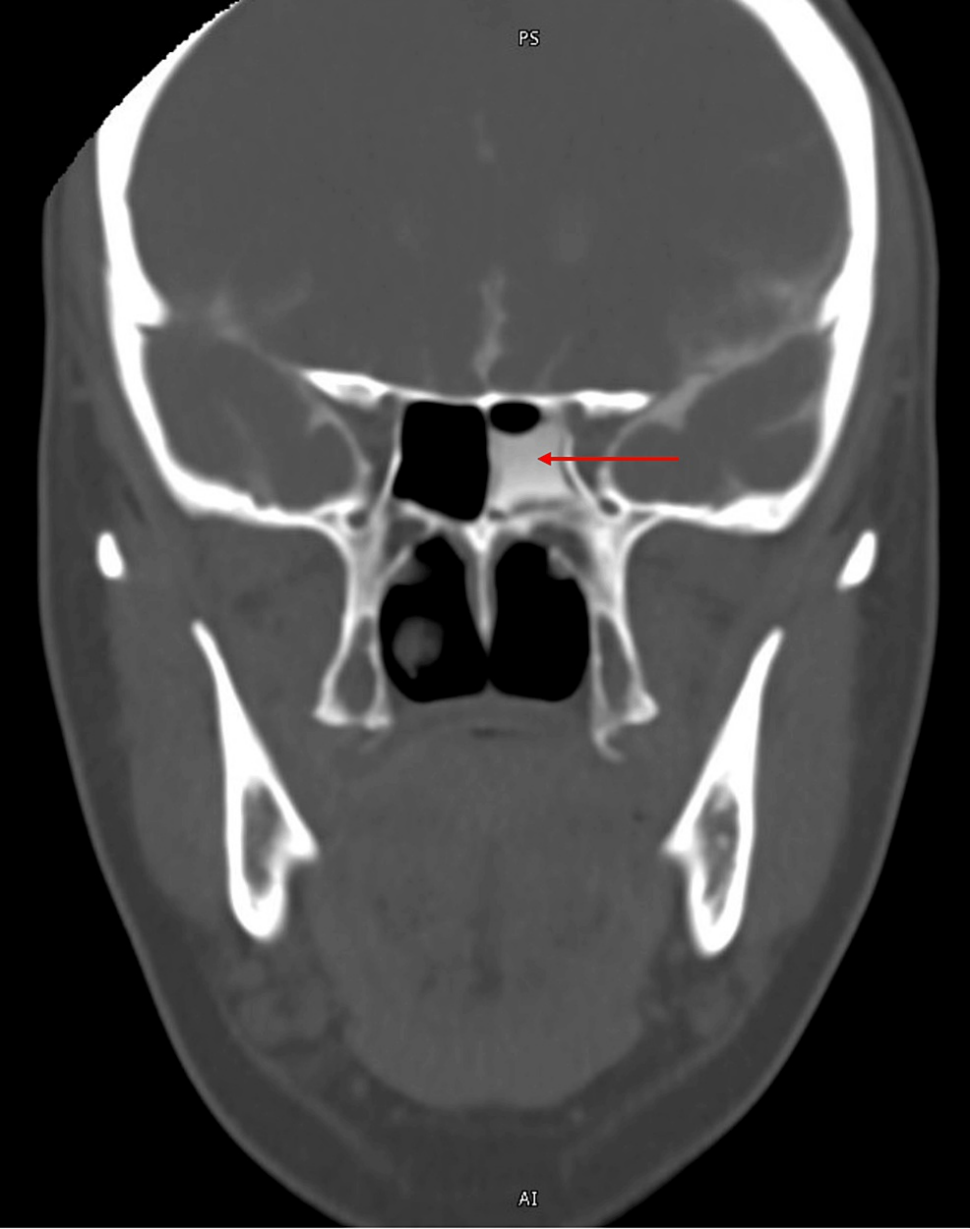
CT cisternogram performed due to a high suspicion of a CSF leak in the CT sinus The red arrow highlights contrast extravasation through a bony defect of the posterior sphenoid sinus.
